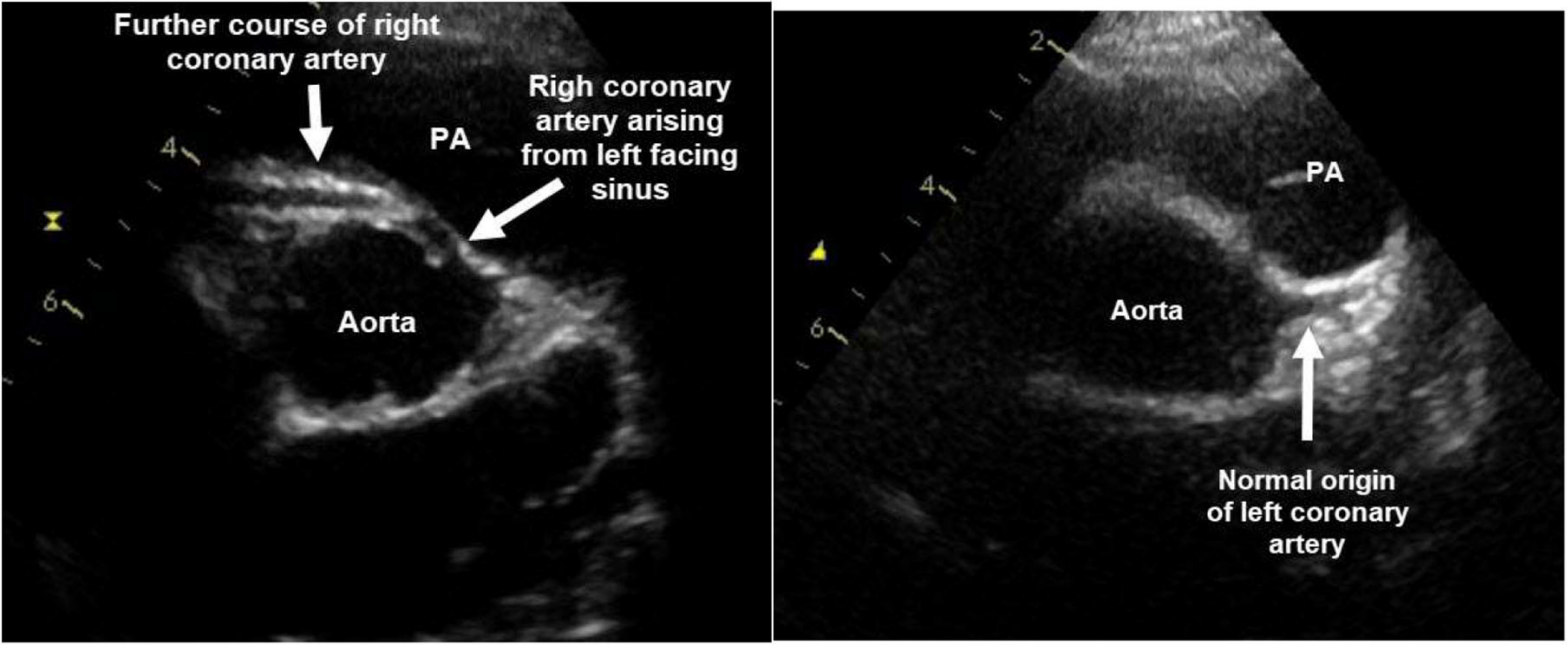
Echocardiography of patient 2: Origin of the right coronary artery (RCA) from the left-facing sinus with an interarterial and intramural course between the aorta and the pulmonary artery (PA). Normal origin of the left coronary artery from the left-facing sinus.
